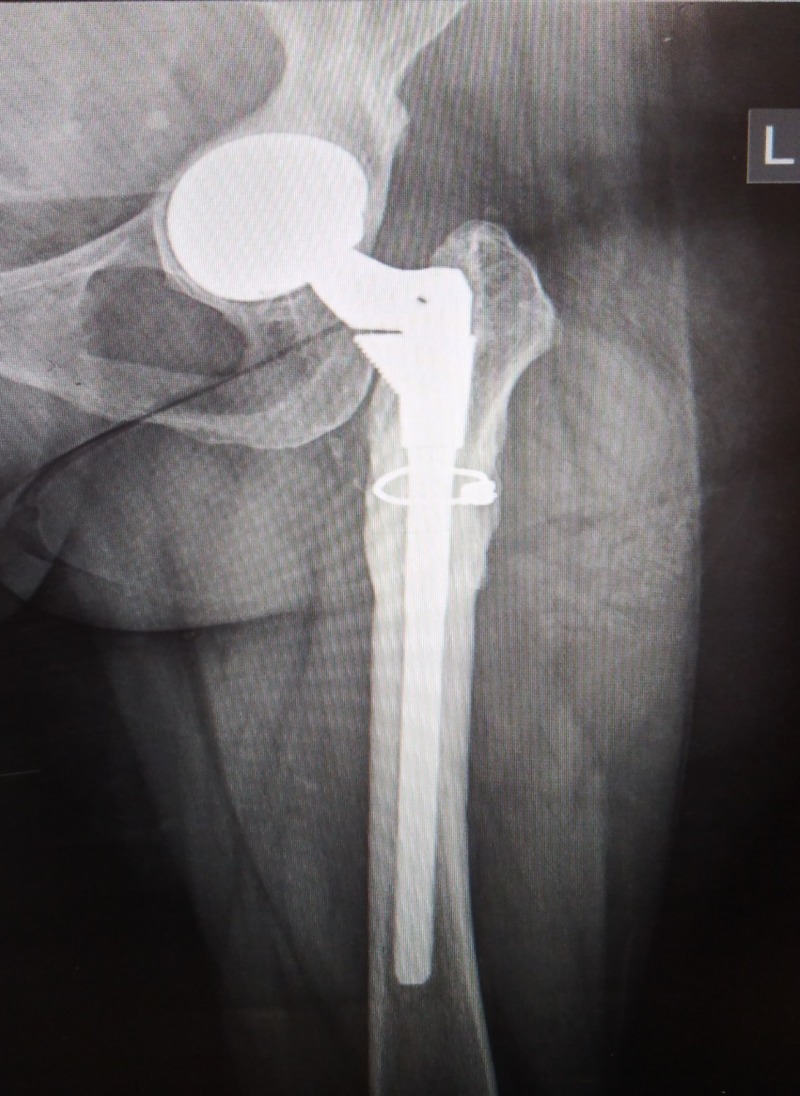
Post-operative X-ray Post-operative modular hip replacement.
